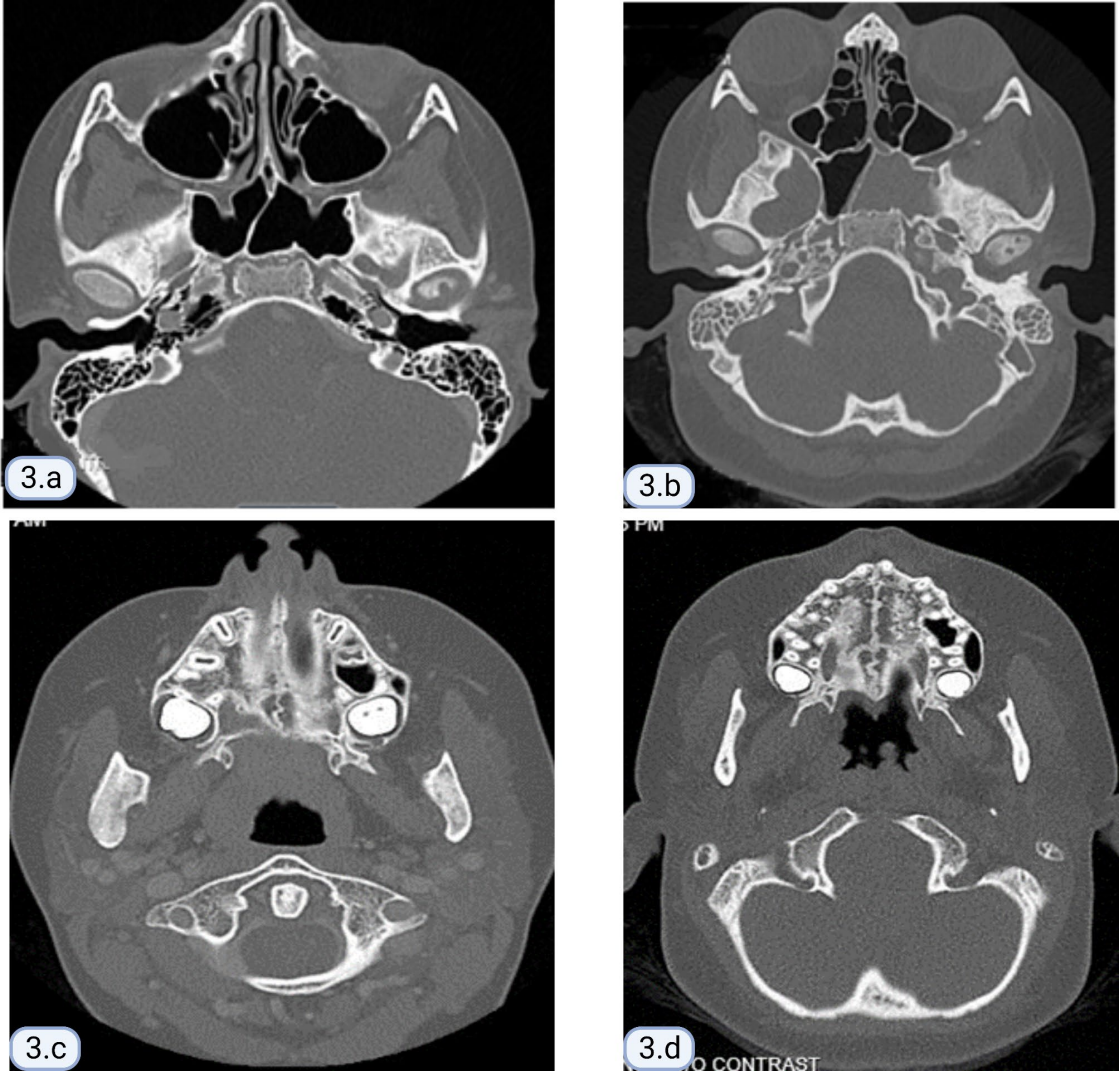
Comparison of CT findings (mastoid air cells-top & mandibular lesion-bottom) between 2018 and 2022. (a) CT showing clear mastoid air cells (2018), (b) CT showing opacification of the mastoid air cells (2022), (c) CT showing expansion of the right mandibular ramus and periosteal reaction (2018), (d) CT showing normal mandibular ramus (2022)
